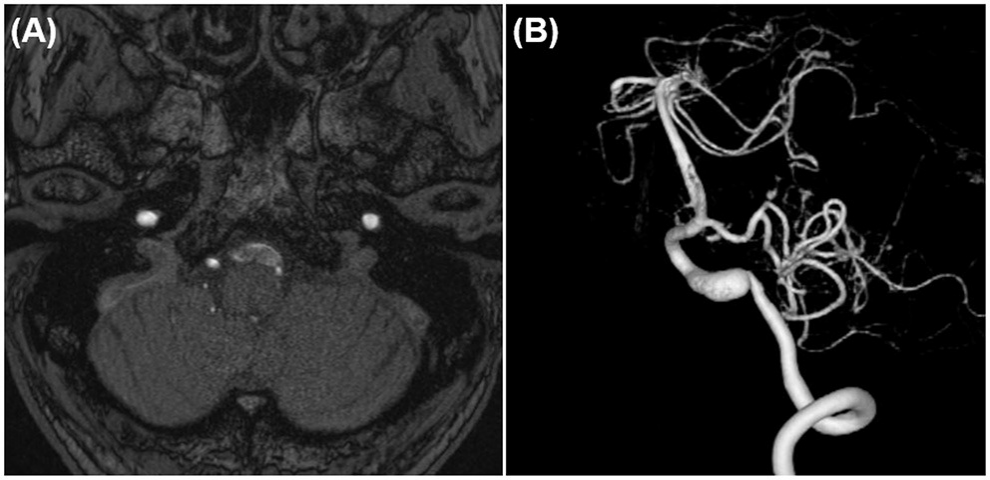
Preoperative image examinations of case 2. (A) Magnetic resonance imaging. (B) Digital subtraction angiography.
